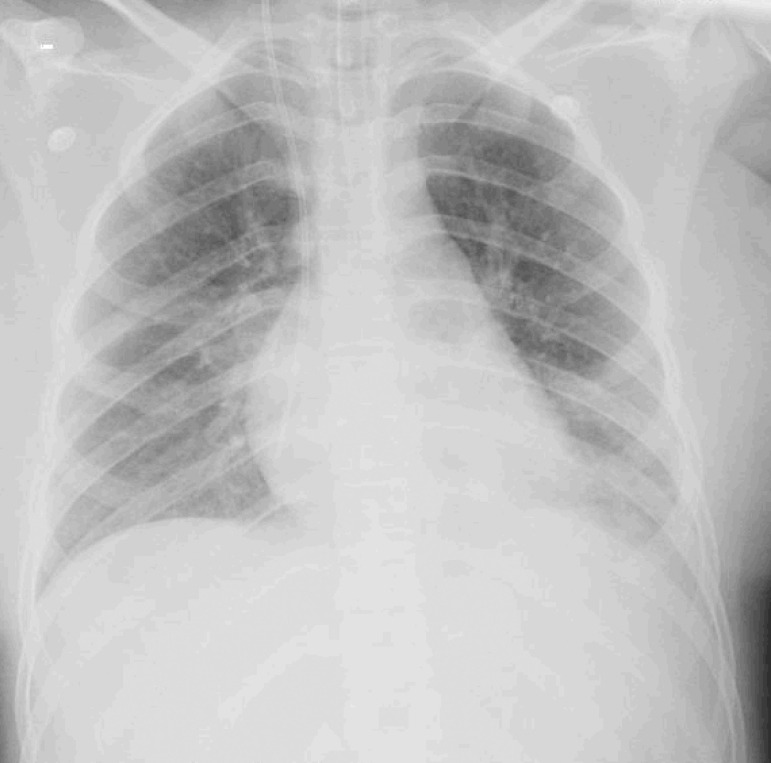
Admission chest radiography of a patient with Influenza B infection and subsequent diagnosis of Kawasaki disease.
